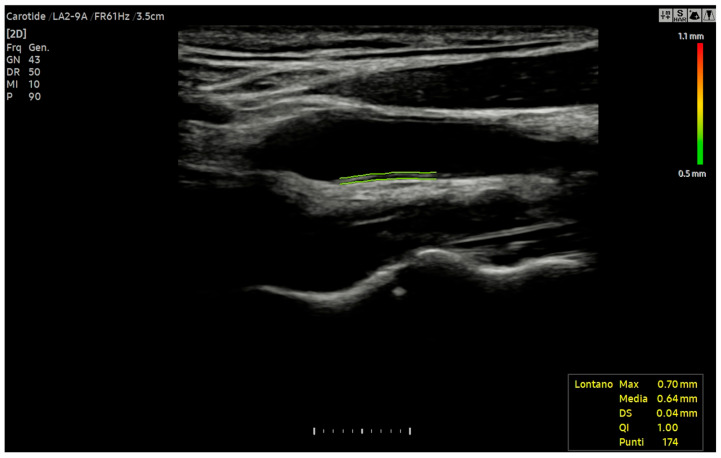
Automatic IMT measurement of far walls of the left common carotid artery using Auto IMT™ software (RS 85, Samsung Medison diagnostic ultrasound system, version 1.05, SAMSUNG MEDISON CO., LTD., 3366, Hanseo-ro, Nam-myeon, Hongcheon-gun, Gangwon-do 25108, Republic of Korea).
